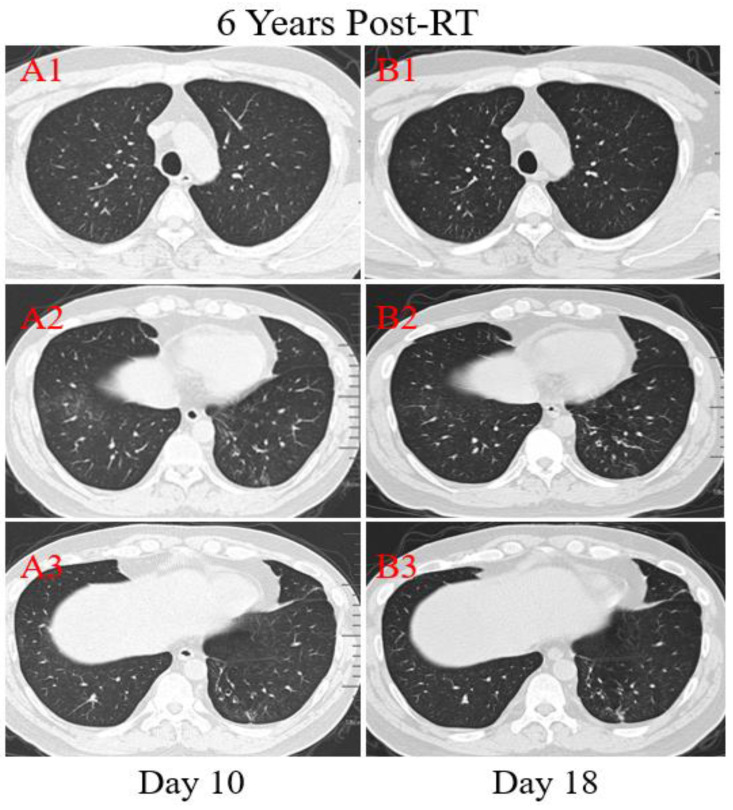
Transverse unenhanced thin-section serial CT scans from a 39-year-old male with COVID-19 pneumonia. Chest CT images on the 10th day after symptom onset demonstrated multiple ground-glass opacities of the lower lobes of both lungs peripherally (A2), and a few linear opacities in upper lobe lower lingual segment of the left lung (A3). Follow-up CT at the 18th day demonstrated significant improvement in the extent and density of the ground-glass opacities (B2), and appearance of new focal ground-glass opacities of the upper lobe of right lung (B1).
